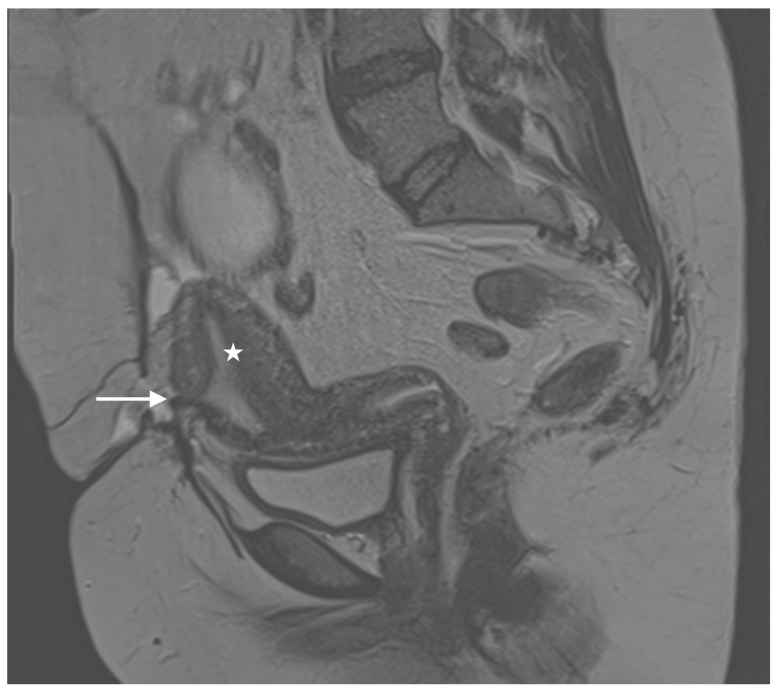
Diagnostic imaging (MRI, T2) shows a scar region (arrow) within the ventral corpus uteri. The corpus uteri (asterisk) is displaced ventrally towards the abdominal wall. In alignment with the clinical symptoms, the findings are highly suspicious for fistula formation.
